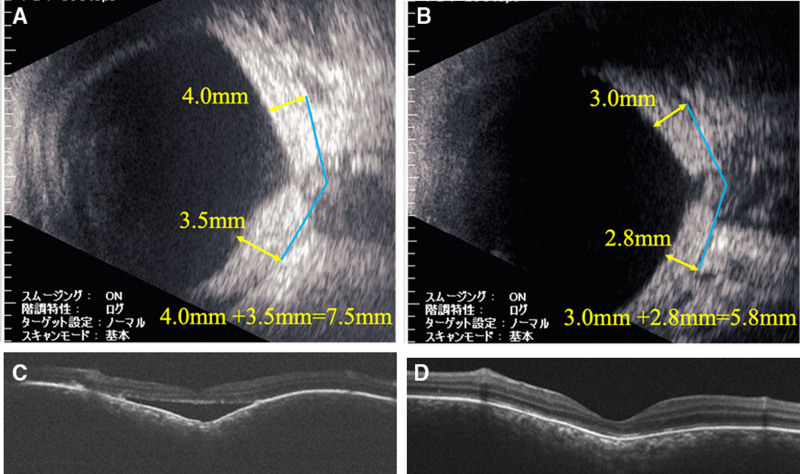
(A) B-mode ophthalmic ultrasound before proton beam therapy in the left eye. The height of the hemangioma was 7.5 mm (4.0 + 3.5 mm). (B) B-mode ophthalmic ultrasound 7 mo after proton beam therapy in the left eye. The height of the hemangioma was 5.8 mm (3.0 + 2.8 mm). (C) OCT before proton beam therapy. Mild retinal detachment was observed only in the macula. (D) OCT 7 mo after proton beam therapy. Retinal detachment improved. OCT = optical coherence tomography.
